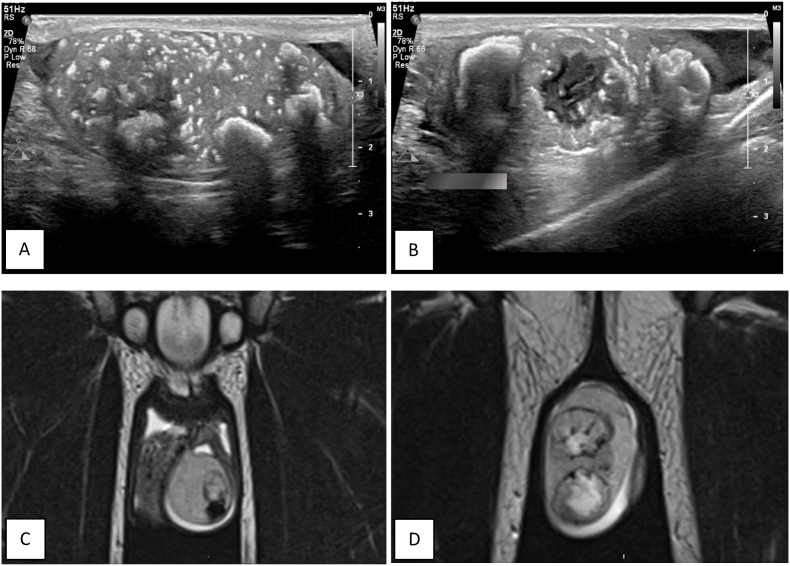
Radiological evaluation, A and B: US showing multiple lobulated heterogenous lesions with extensive calcifications bilaterally, C and D: MRI scan showing multiple bilateral testicular masses with calcifications and cystic changes without extra testicular extension.
